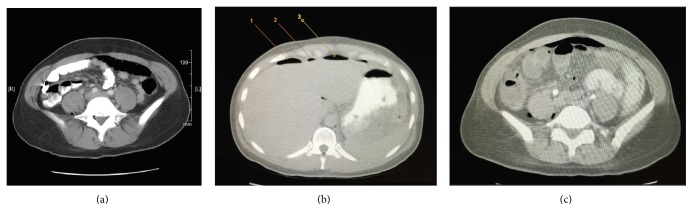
Computed tomography (CT) of the abdomen/pelvis demonstrates the progression of intra-abdominal injury and free air accumulation. (a) Transaxial view highlighting the accumulation of air in the spinal canal, psoas muscles, and the retroperitoneum, secondary to deep penetration and injury of the small bowel during the laminectomy. (b) This axial view demonstrates three distinct locations (labeled 1, 2, and 3) of intra-abdominal free air found on a follow-up CT of the abdomen/pelvis. (c) Axial view depicting extraluminal air anterior to the bowel, as well as air within the mesentery and posterior to the right psoas muscle.
